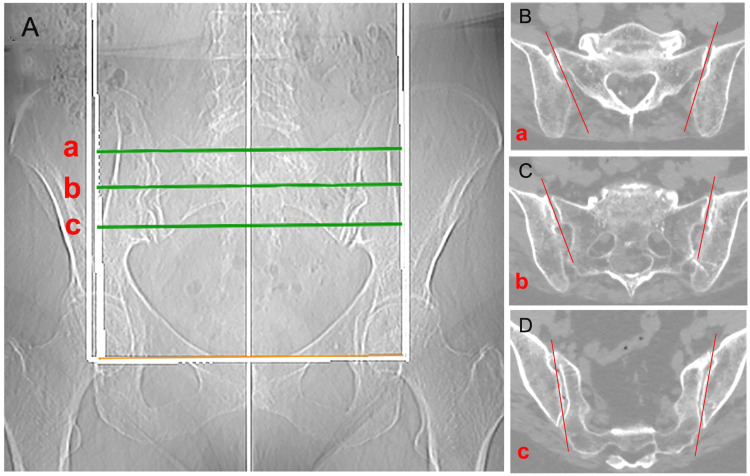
CT scan of the sacroiliac joints. In the coronal view (A), the green lines show in the axial cuts; a) upper (B); b) middle (C); and c) lower (D) portions. The red lines highlight obliquities of the axes of the articular surfaces. Image Credit: Author Saverio Colonna
